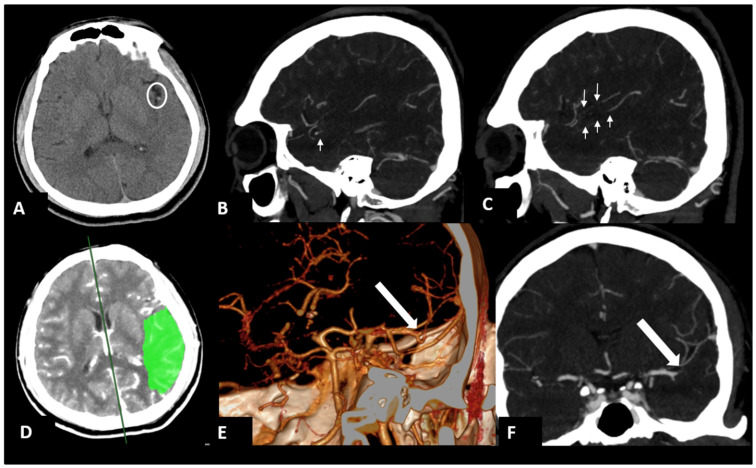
Brain computed tomography (CT) angiography in the ER. The images showed a high-density vessel sign at the left middle cerebral artery (MCA) on axial non-contrast CT (circle in (A)) and filling defects in left M2–M3 branches of MCA (arrows) on sagittal maximum intensity projection (MIP) images (B,C) and volume rendering (E) and coronal MIP images (F). CT perfusion revealed a large penumbra (green) in the left temporoparietal lobes (D).
